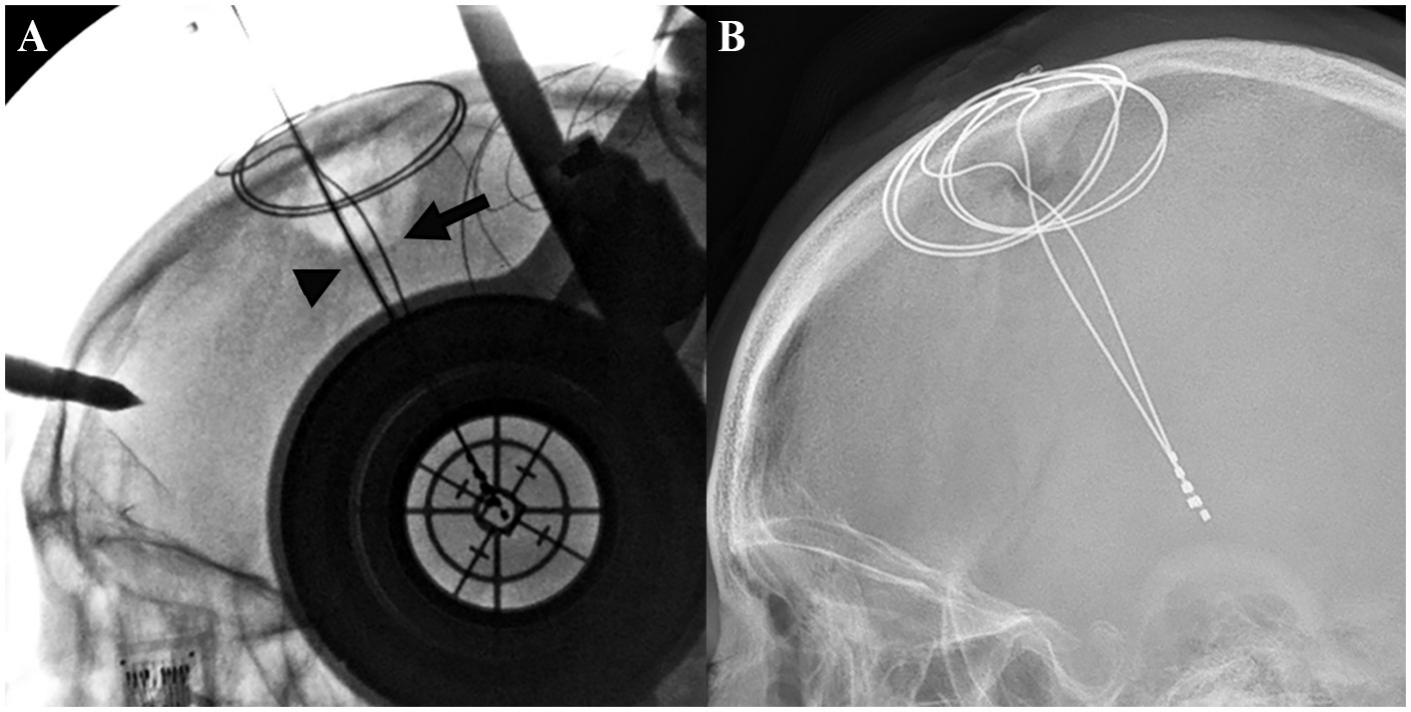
Timing of bending occurrence. A. When the implanted lead is still secured in the guide tube, the lead is straight (arrowhead). In contrast, another lead which is permanently implanted and secured by the burr hole cover is already bent (arrow). B. Immediate postoperative lateral skull X-ray of the identical patient shows that both leads are bent.
